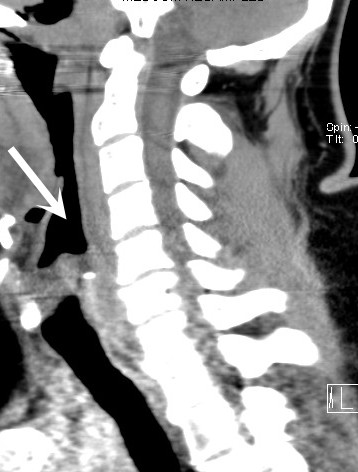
Sagittal reconstruction from a CT scan upon patient arrival shows mild prominence of the prevertebral soft tissues, without clinically significant hematoma or encroachment on the airway (large white arrow).
